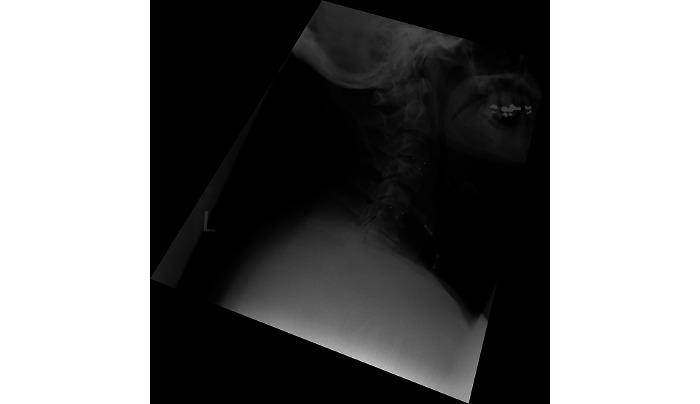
Postoperative x-ray of the cervical spine.
